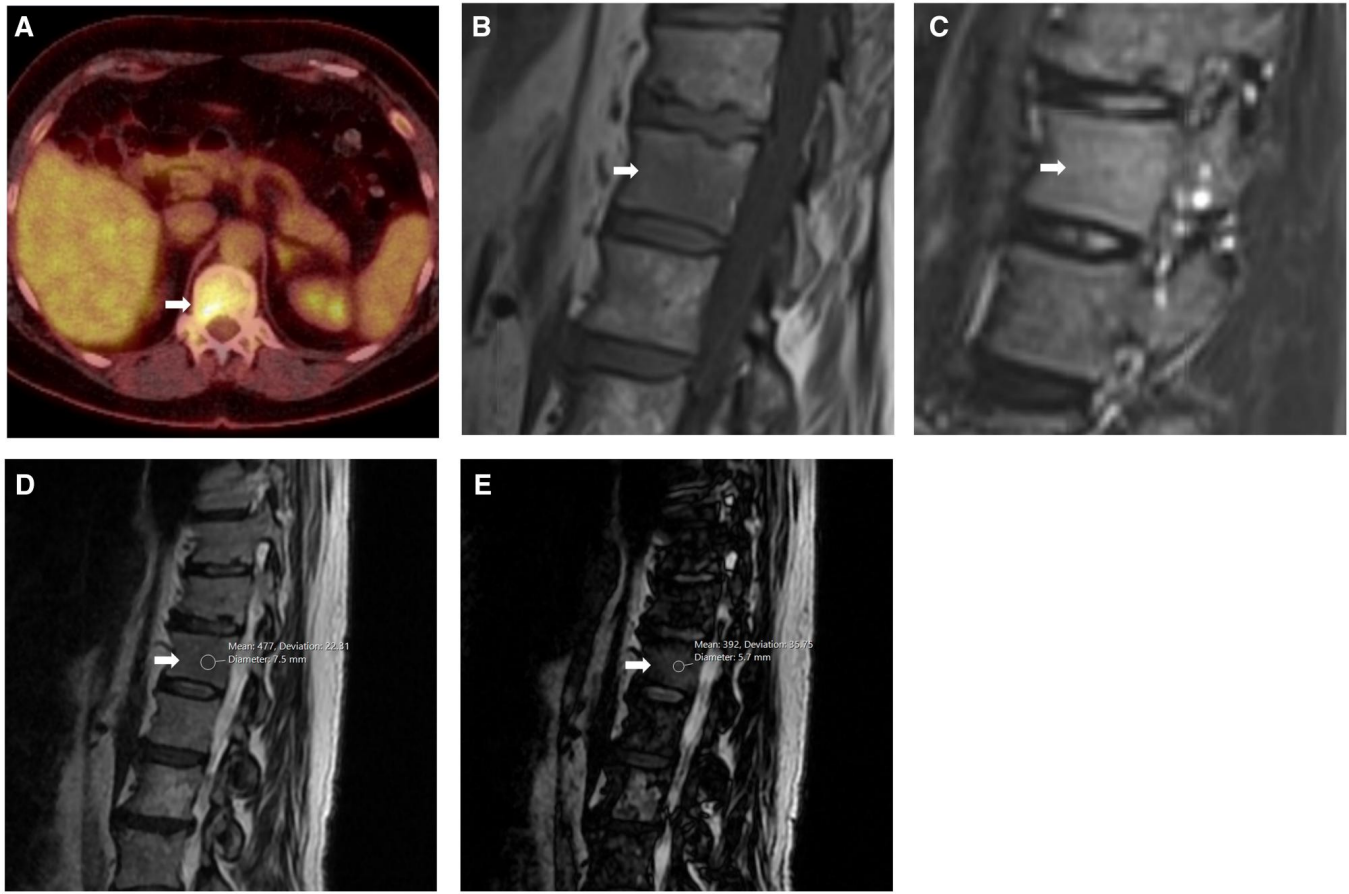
T12 vertebral body lesion (white arrow) in a 68-year-old female undergoing investigation for suspected lung malignancy. (A) Axial FDG PET images demonstrate a focally avid lesion with an SUV of 5.3. (B, C) Sagittal MRI sequences performed 10 days later demonstrates ill-defined reduction in T1w (B) with a subtle increase in STIR (C) signal. (D, E) Sagittal Dixon sequences showed no significant drop-out on in (D) and out (E) of phase imaging. Repeat MRI 6 months later showed stable imaging characteristics.
